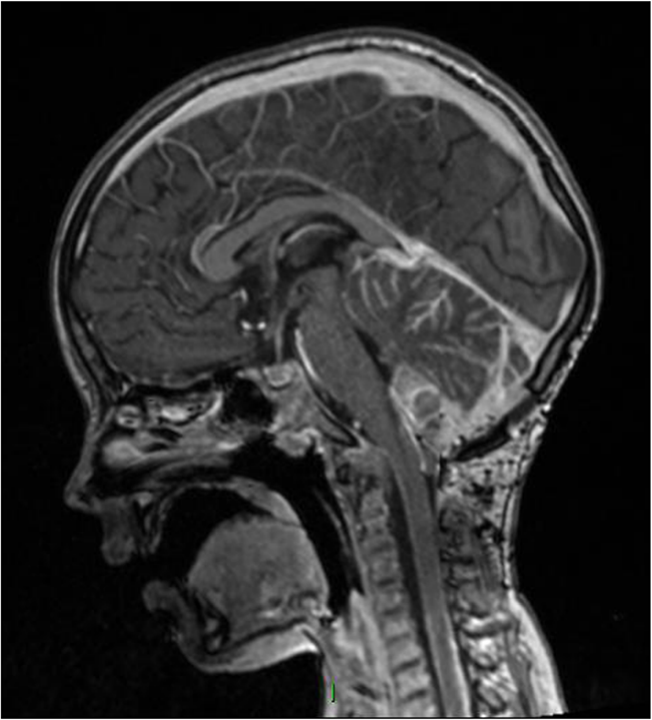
MRI 49 days post-resection
